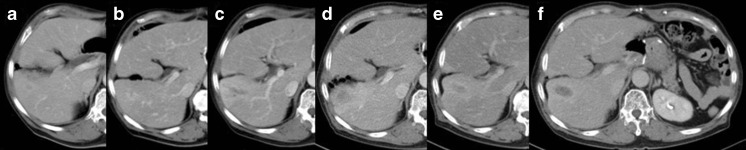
The changes in the CT images during the 5 years of observation. a June 2006. b May 2007. c July 2008. d Aug 2009. e Oct 2010. f July 2011
